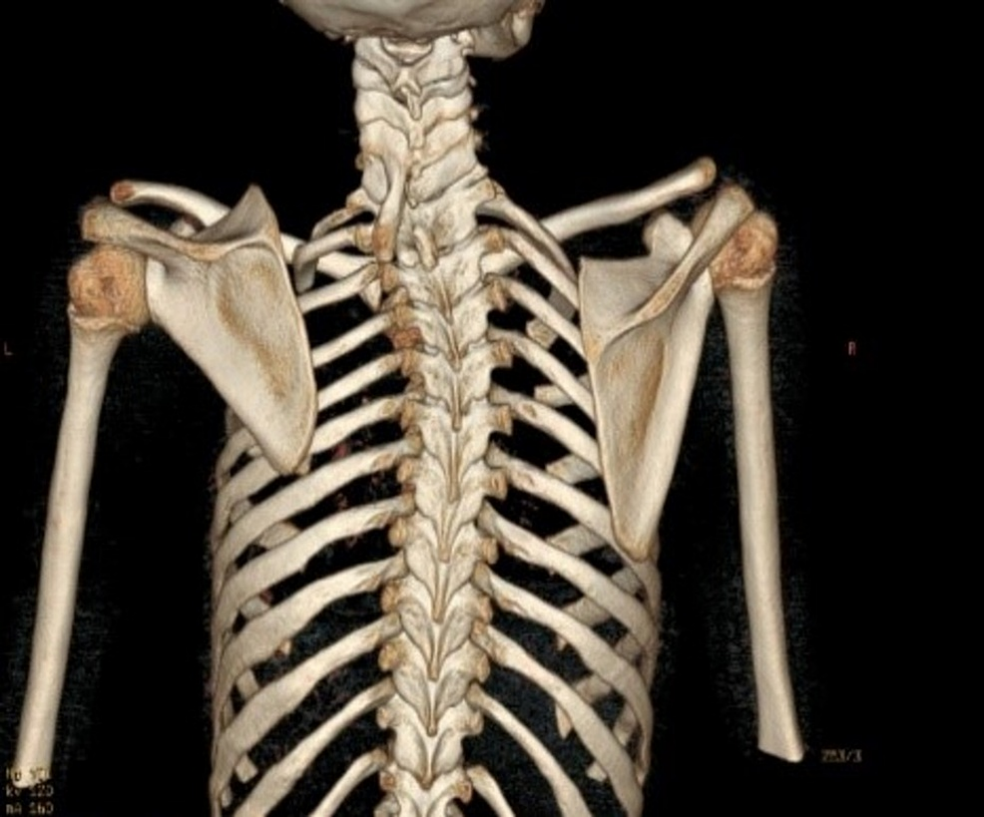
CT scan of the patient showing congenital scoliosis with unsegmented bars and elevated left scapula CT: computed tomography
